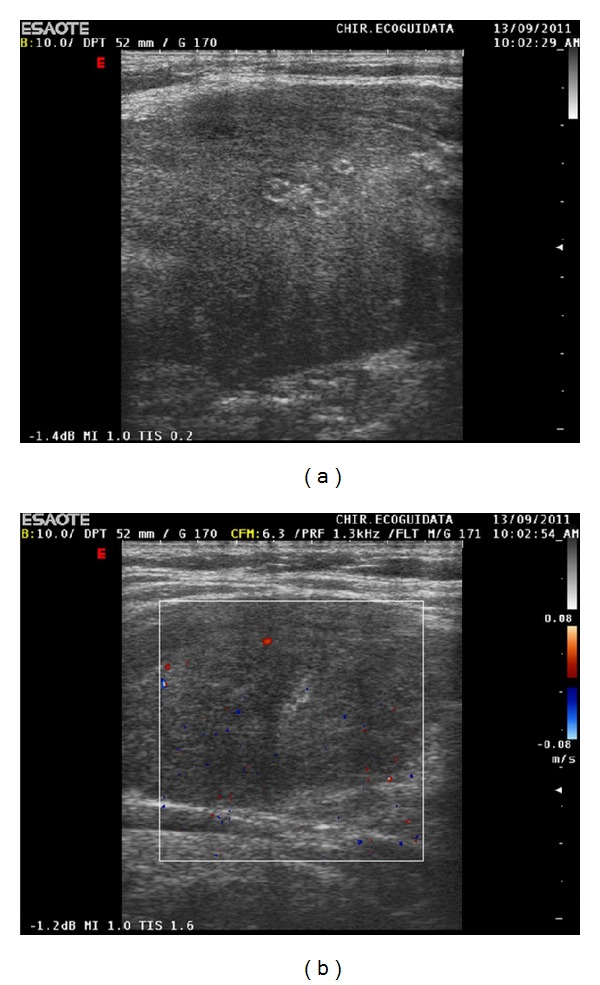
Ultrasound examination. (a) Longitudinal scan of the thyroid right lobe; the gland echogenicity is similar to that of the strap muscle. The postablative laser scares are clearly visible as “target like” focal areas inside the gland. (b) The color Doppler examination reveals a poor or absent vascular pattern.
